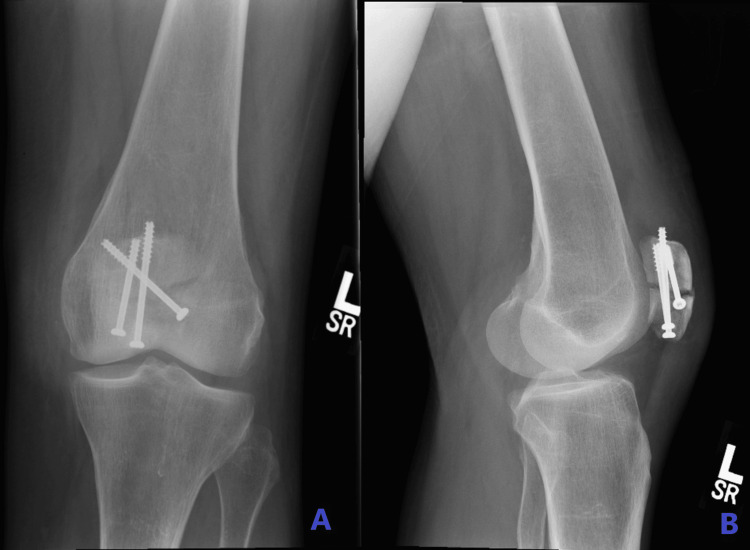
Plain antero-posterior (A) and lateral (B) X-rays after open reduction internal fixation of a transverse fracture of the patella
